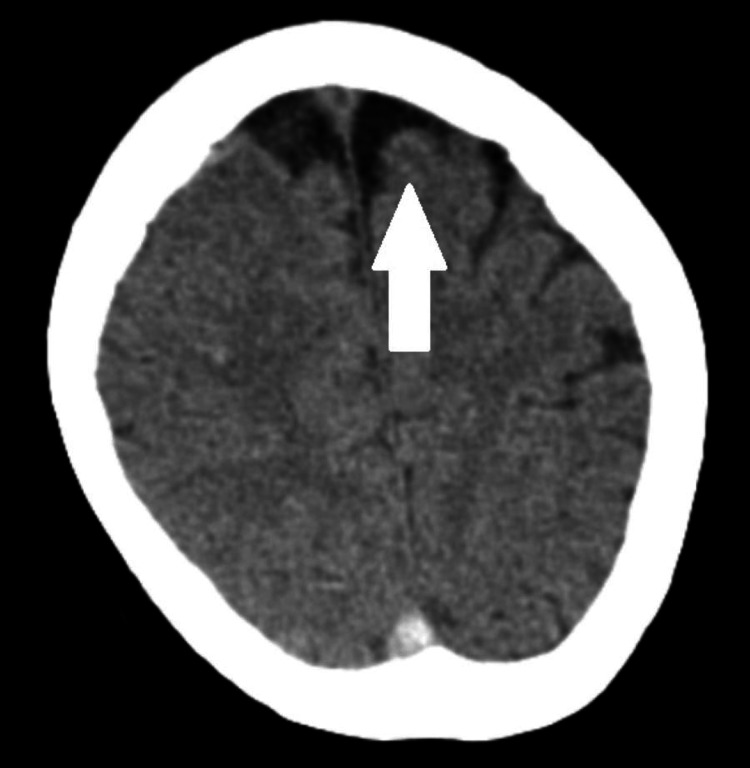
Computed tomography scan showing ill-defined area of hypodensity
